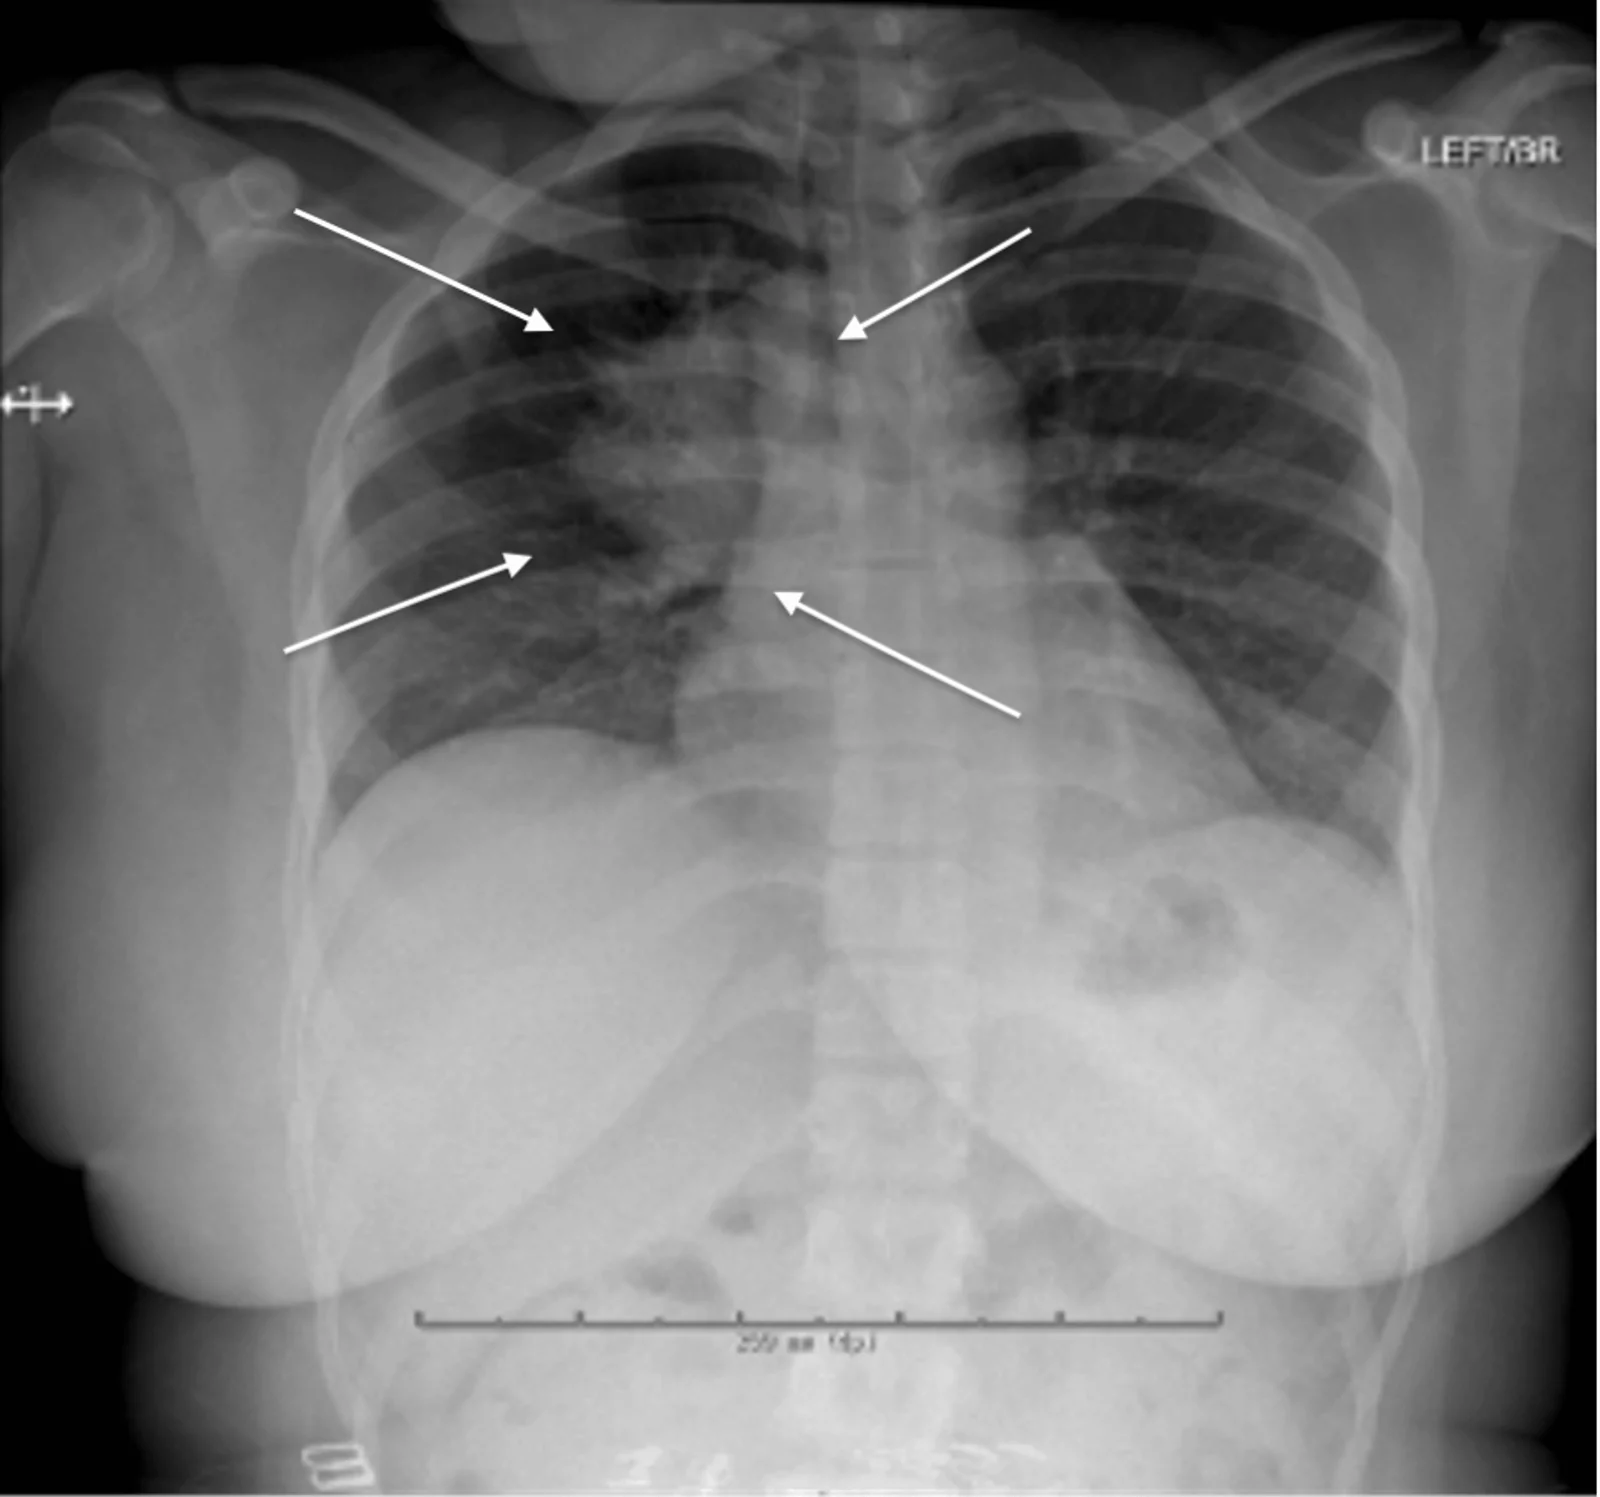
Initial chest X-ray (anteroposterior view). Demonstrating a right hilar opacity concerning focal pulmonary consolidation. Arrows point to the right hilar opacity.
